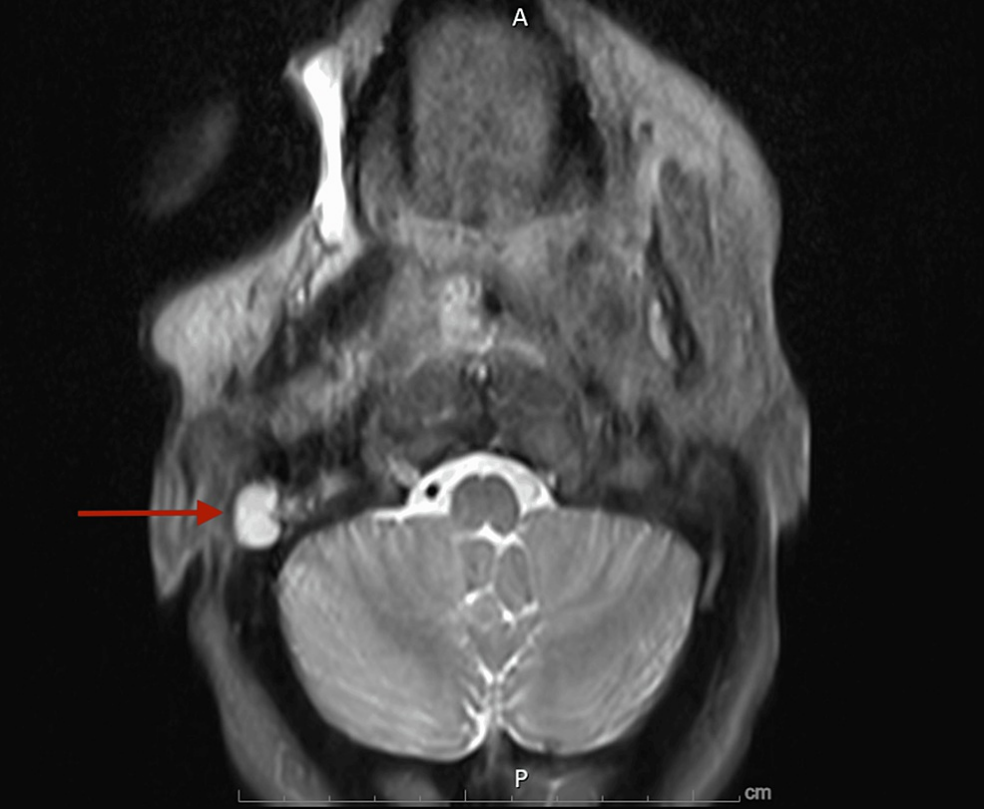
MRI of the orbit, face, and neck with contrast Seen is an avidly enhancing 3.7 cm mass within the right middle ear and mastoid air cells (red arrow) indicating a glomus tympanicum.
